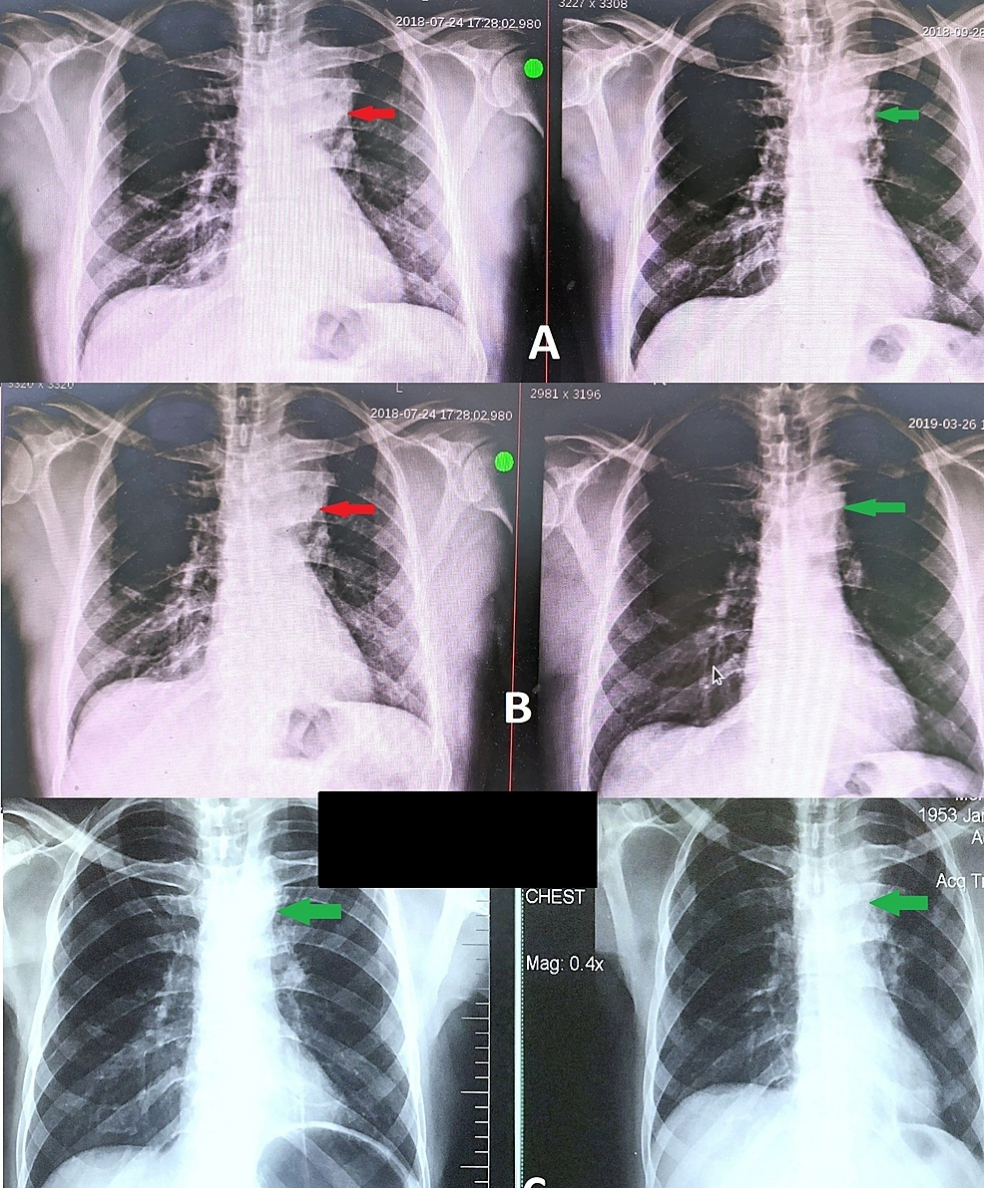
Chest X-ray images. A: Round ill-defined opacity in the mediastinum (red arrow) and reduced opacity size post-anti-tuberculous therapy initiation (green arrow). B: Recurrence of mediastinal opacity two years later (red arrow) and resolution of opacity post-mycophenolate mofetil (MMF) initiation (green arrow). C: Resolved opacity immediately post-MMF initiation (green arrow) and sustained resolution till now (green arrow).
